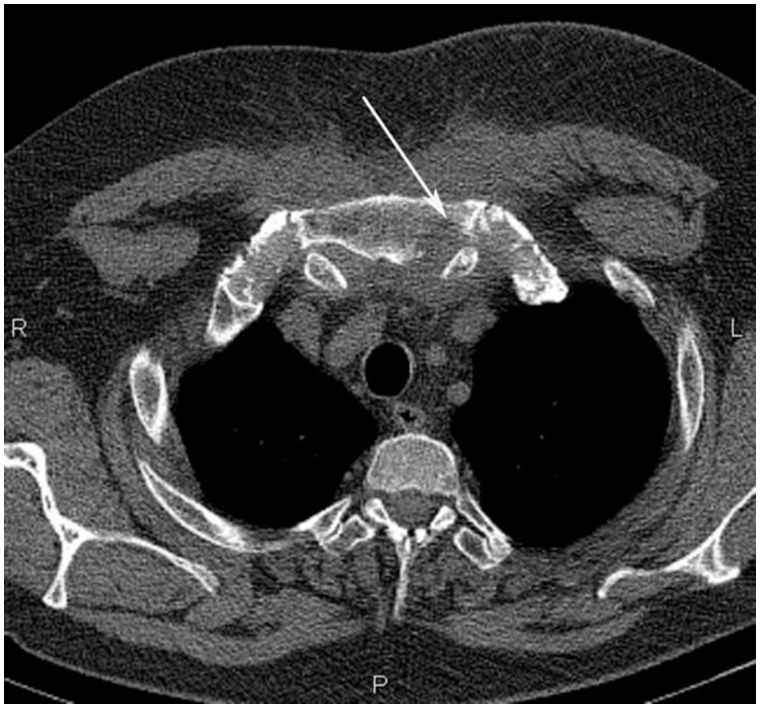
Transverse plane computed tomography image showing signs of destruction of the left sternoclavicular joint (arrow)
